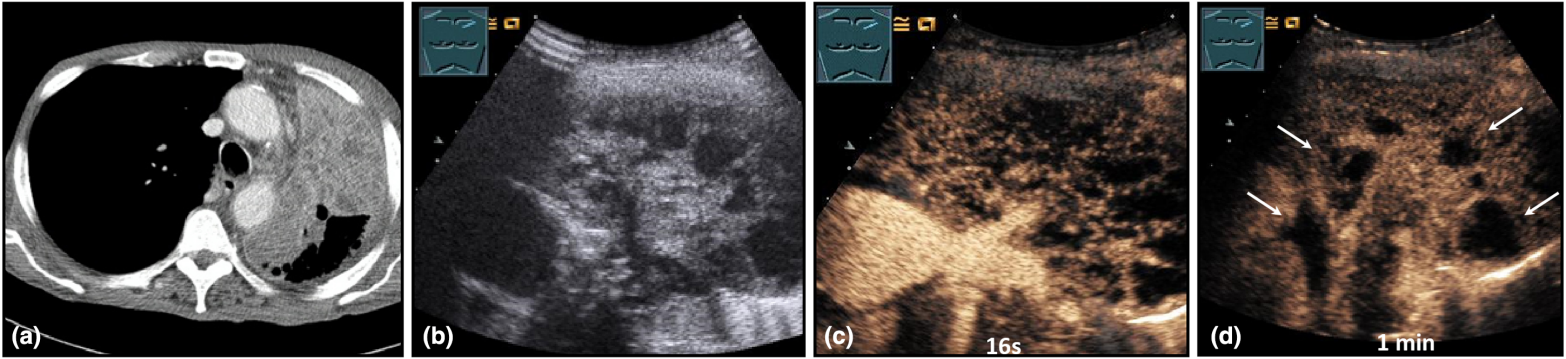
A 70‐year‐old patient presented with fever, weight loss, shortness of breath, and cough with purulent sputum due to left‐sided bronchial carcinoma. Visualisation of a left‐sided, central tumour formation with subsequent inhomogeneous consolidation on computed tomography (courtesy of Prof. Dr. Andreas H. Mahnken, Department of Radiology, University Hospital Marburg) (a); B‐mode ultrasound shows a hypoechoic, inhomogeneous consolidation (b); contrast‐enhanced ultrasound reveals pure bronchial arterial perfusion after 16 seconds (c). After 1 minute (d), anechoic, round lesions (arrows) demark, as in obstructive purulent pneumonitis. Bronchoscopic transtumoral recanalization was not successful.
